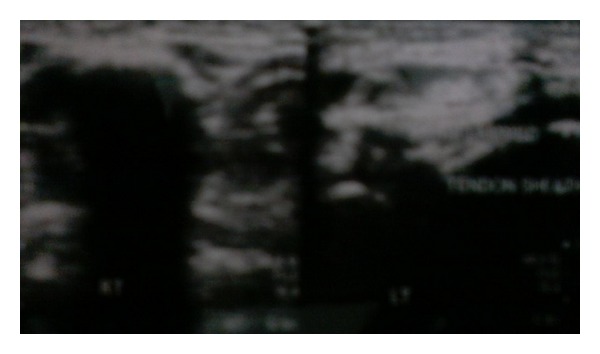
Color Doppler of limb showing inflamed tendon sheaths.
